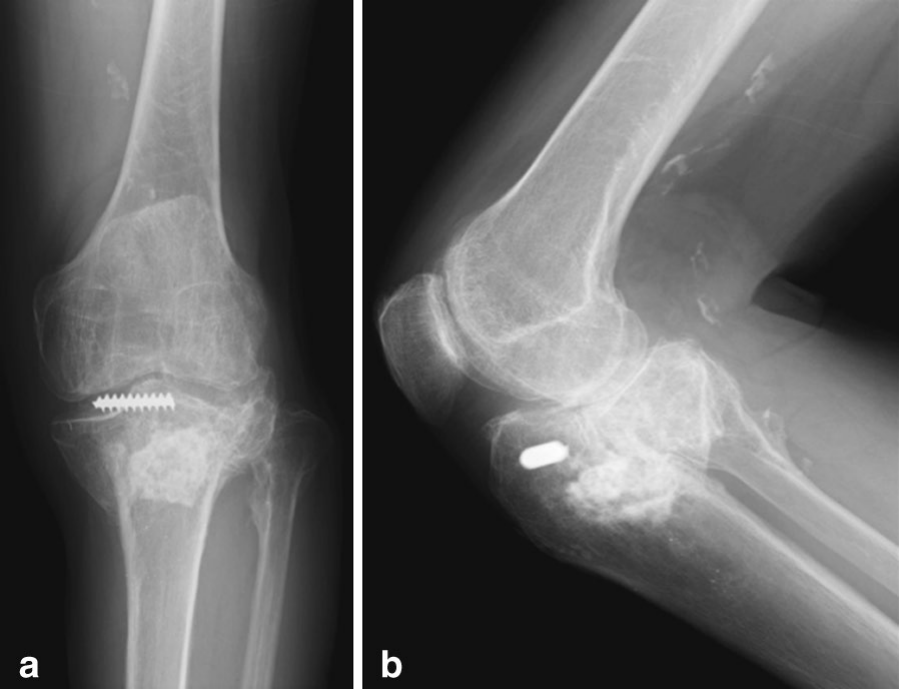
Radiographs of the left knee. a Anteroposterior view shows a shortened proximal end of the tibia due to the fracture and varus deformity. b Lateral view shows a collapsed articular surface of the posterior tibia with marked posterior angulation
